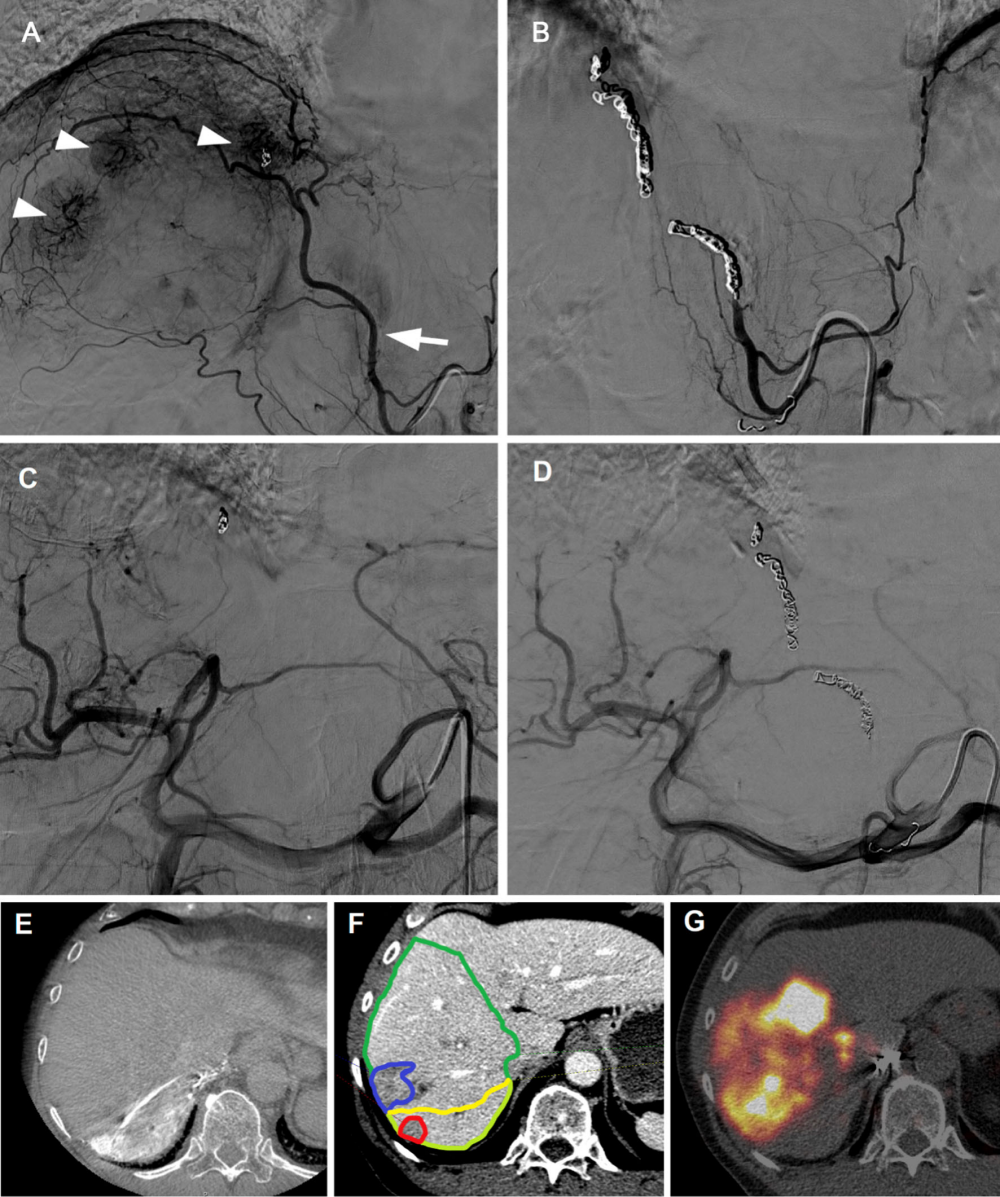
Example of poor redistribution. A DSA showing parasitized blood supply to several liver tumors (arrow heads) from the right inferior phrenic artery (arrow). An old microcoil from a prior procedure in another hospital is also visible. B Coil embolization of the phrenic artery. C Celiac trunk DSA prior to coil embolization of the phrenic artery. D Celiac trunk DSA post-coil embolization. E Cone-beam CT of the right phrenic artery shows enhancement of tumors in segment VII (prior to coil embolization). F Volumes of interest drawn in Simplicit^90^Y software; the liver volume supplied by the phrenic artery segment was delineated using cone-beam CT data. G^90^Y-PET/CT after injection of ^90^Y-microspheres in the right hepatic artery shows no redistribution to segment VII
